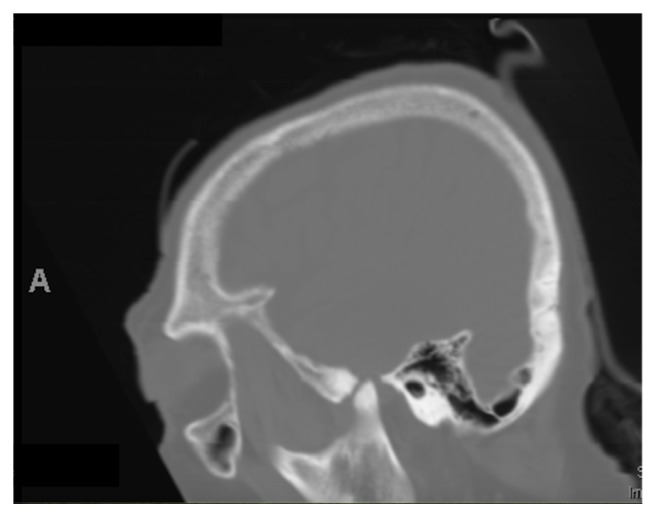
Sagittal CT demonstrates erosion of the skull base and protrusion of the TMJ.
